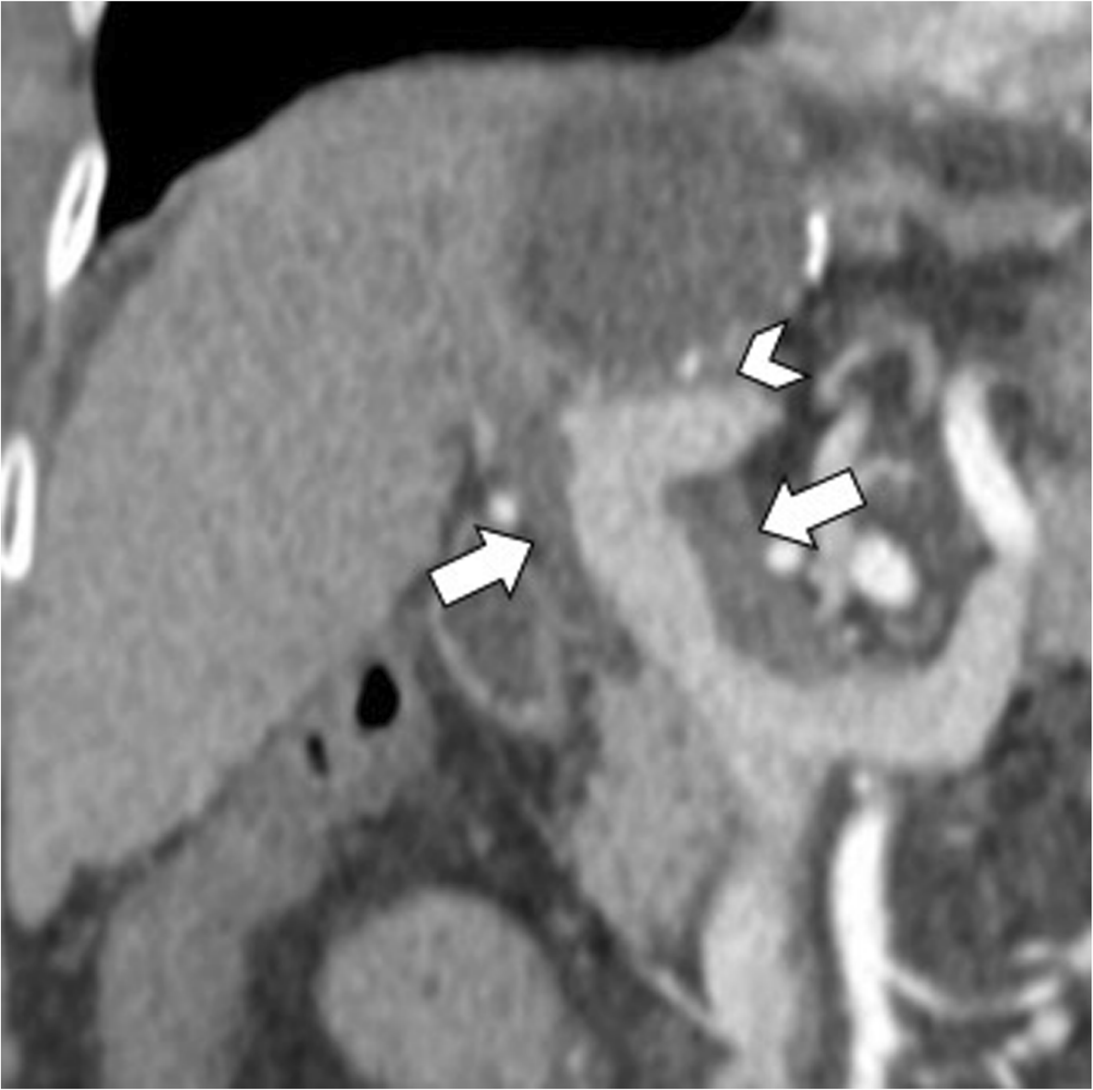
A 72-year-old woman with hydatid cyst and portal vein thrombosis. Oblique reformatted CT image on portal venous phase shows a large hepatic hydatid cyst adjacent to the left branch of portal vein (arrowhead) and partial portal vein thrombosis (arrows)
